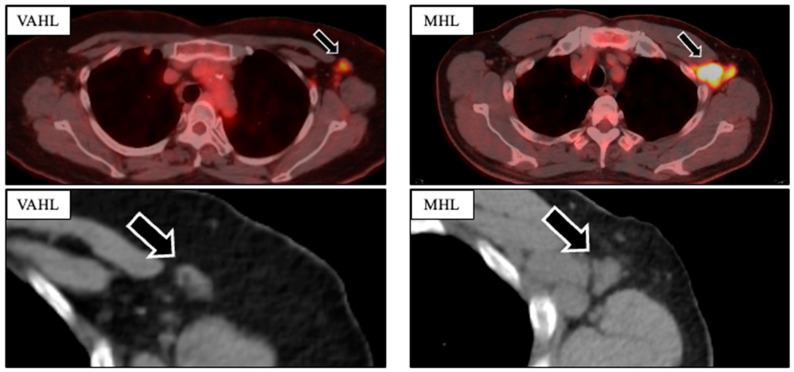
Comparison of PET-CT imaging findings in VAHL and MHL to assess the morphology (minor axis, major axis, presence of fatty hilum) and ^18^F-FDG uptake intensity of the axillary lymph nodes.
